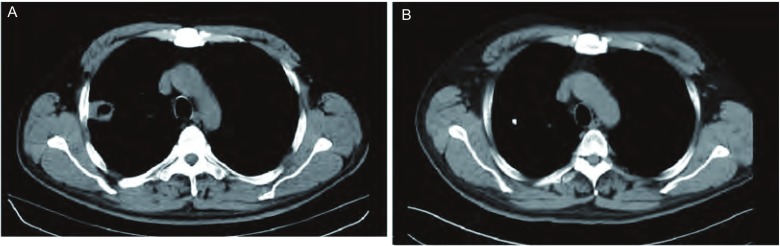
射波刀治疗前和治疗后2个月的胸部CT比较。A：射波刀治疗前的胸部CT；B：射波刀治疗后2个月的胸部CT。 Comparison chest CT before CyberKnife treatment with that at 2 months after treatment. A: Chest CT before CyberKnife treatment; B: Chest CT at 2 months after treatment.
